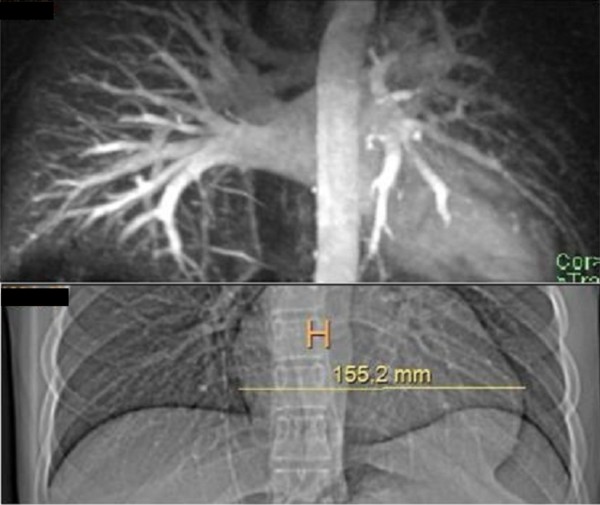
Computed tomography (CT) of the thorax revealed prominent cardiomegaly and pulmonary vessels.
